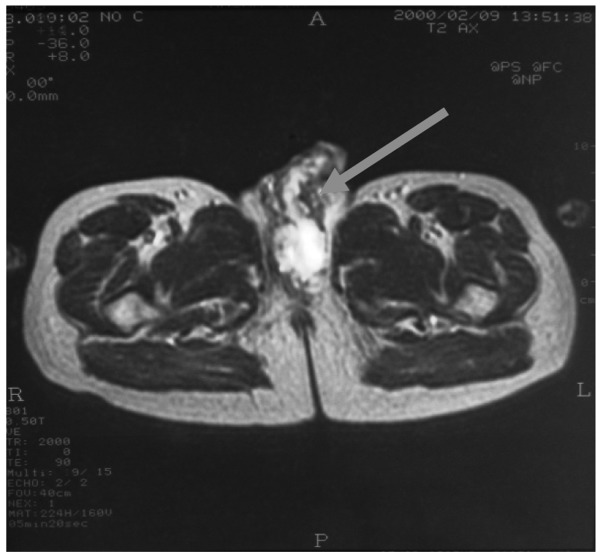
Magnetic resonance imaging revealing a mass in the left cavernous body (arrow).
